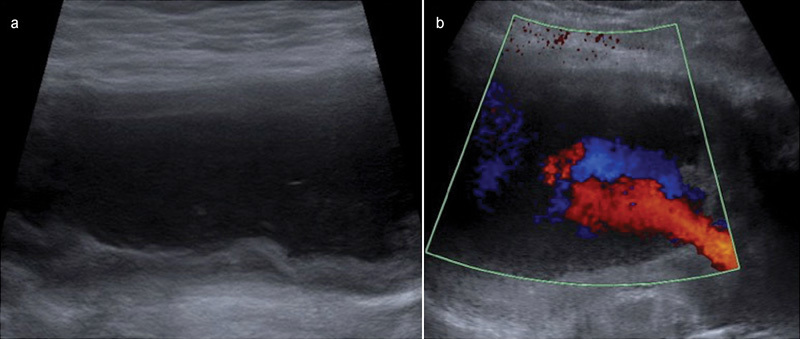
Transverse ultrasound image more posterolaterally (a) demonstrates a second thick-walled cystic structure. Transverse Doppler image (b) demonstrates turbulent blood flow producing a yin yang pattern characteristic of a pseudoaneurysm.
